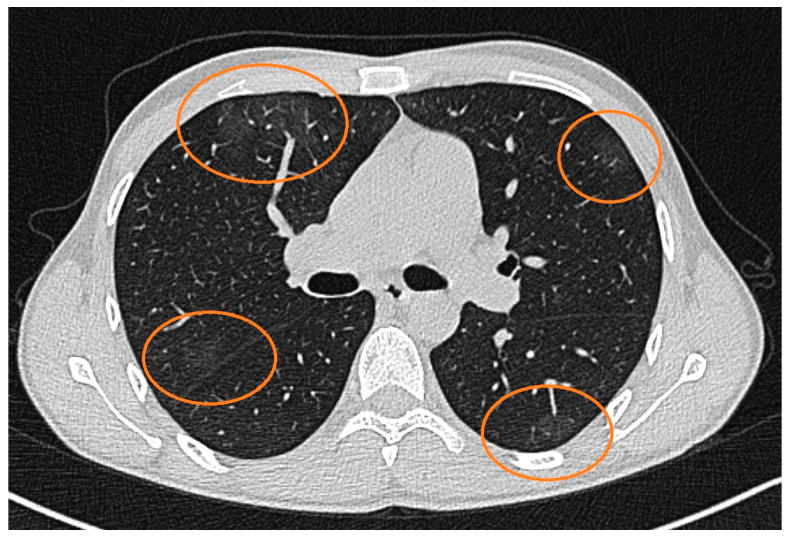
CT scan of the chest showing axial cross-sectional images of the lungs with multiple areas marked in orange circles. These marked areas represent ground-glass opacities. The opacities are distributed in both lungs and subpleural zones, suggesting a diffuse, bilateral pattern. The findings indicate a mild form of SARS-CoV-2 infection, with all lung lobes being affected.
